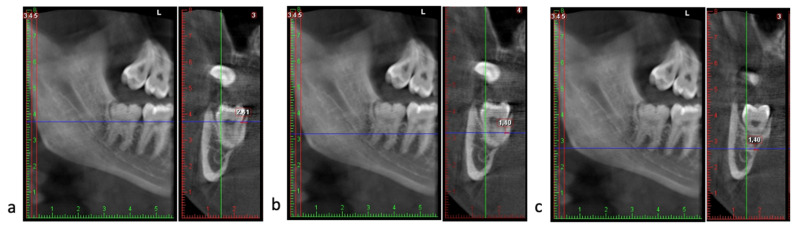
Lingual cortical bone thickness assessment on parasagittal CBCT slices at three levels: (a) cementoenamel junction of the second molar; (b) mid-root level of the third molar; (c) apex of the third molar. Root apices and the lingual cortex are indicated.
